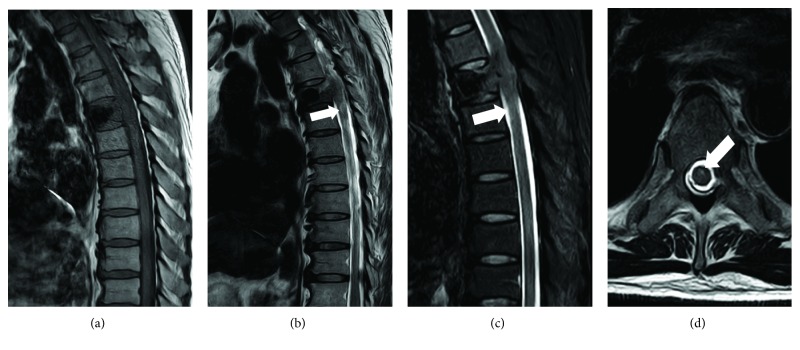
(a) T1-weighted image shows that the T4 body was filled with PMMA and ^125^I seeds. (b–d) The high signal intensity of the spinal cord in T2-weighted and fat-suppression images in the T5 level. The white arrow shows the high signal intensity of the spinal cord.
